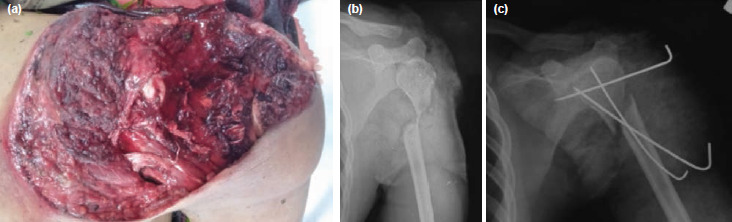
(a) The patient sustained a severe crush injury of the left shoulder. (b) Plain radiograph showed a fracture at the proximal part of the left humerus. (c) After a thorough debridement, the left humerus was temporary held with three 1.6mm Kirschner wires.
